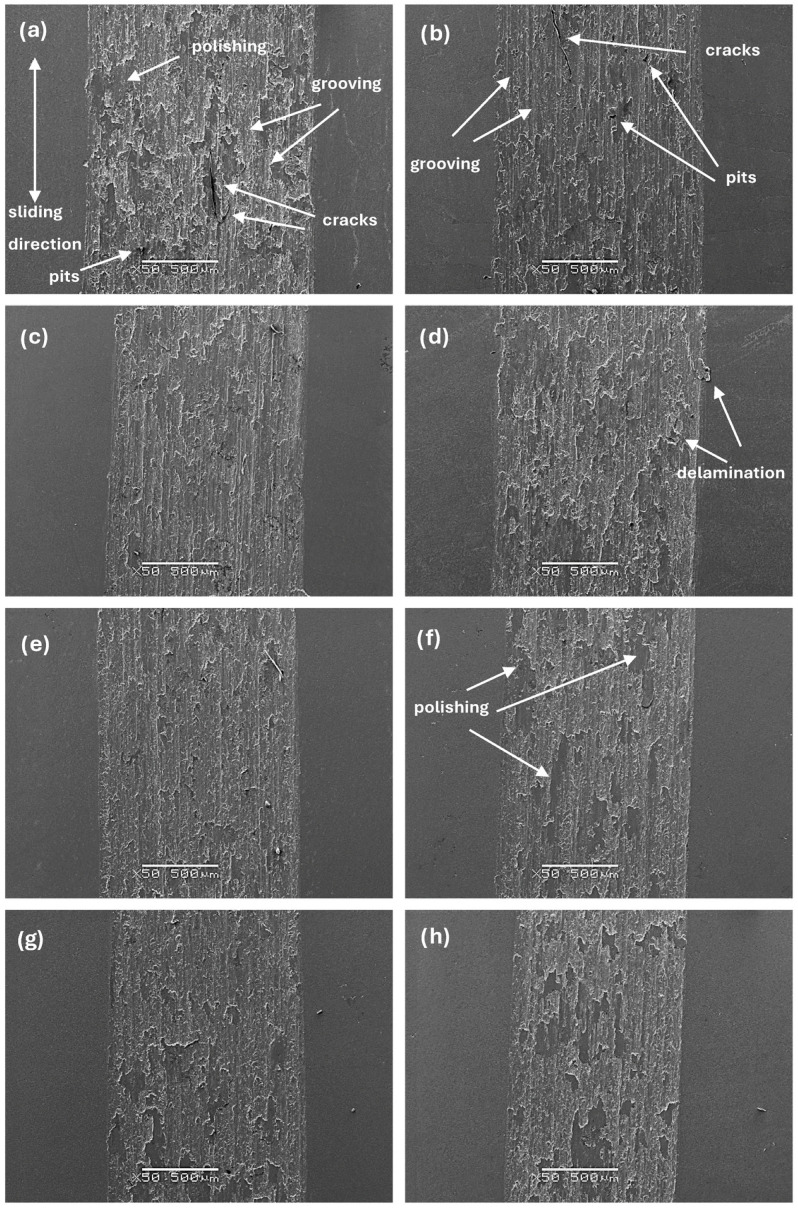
SEM images of wear tracks after ultrasound cleaning for samples: (a) Zr-10Nb, (b) Zr-10Nb-2Mo, (c) Zr-10Nb-4Mo, (d) Zr-10Nb-6Mo, (e) Zr-10Nb-8Mo, (f) Zr-10Nb-10Mo, (g) Zr-10Nb-12Mo, and (h) Zr-10Nb-14Mo.
